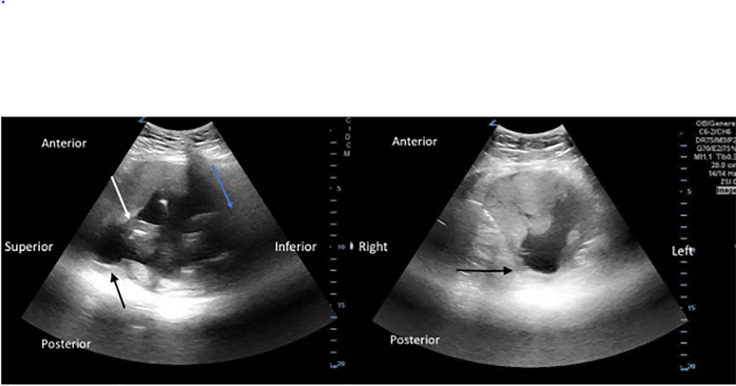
A point-of-care transabdominal obstetric ultrasound in the sagittal and transverse views, respectively. The black arrow demonstrates a defect with thinning of the uterine wall compatible with a uterine sacculation in the uterine fundus. The white arrow indicates fetal parts within the uterus. The blue arrow points toward the inferior portion of the uterus. There was no free fluid surrounding the uterus to suggest uterine rupture at this point.
